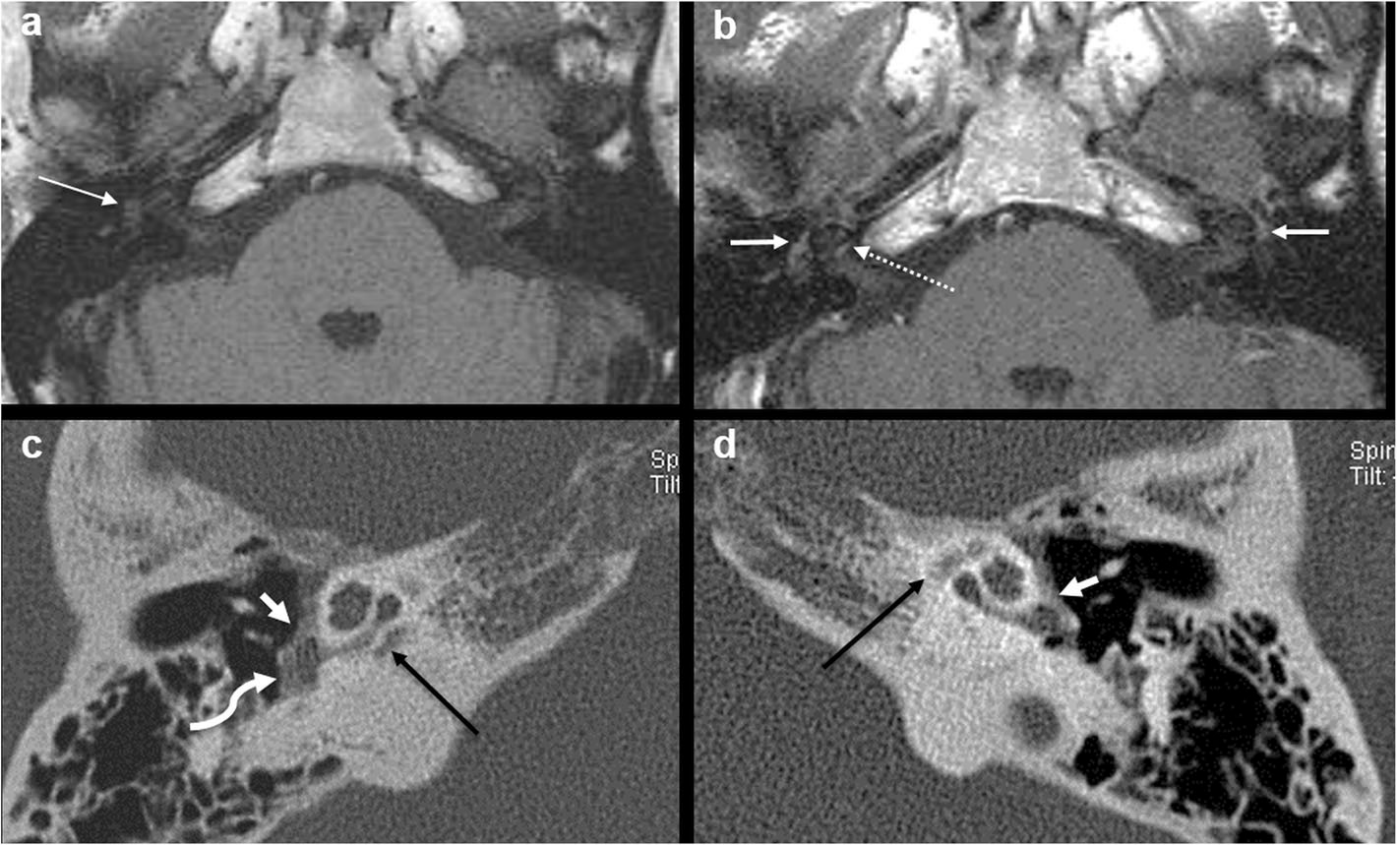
A 41-year-old male patient with left-sided SNHL (case 4). Axial T1W MR image (a) shows intermediate T1 signal in the right FA (arrow). Contrast-enhanced T1W MR image (b) shows moderate enhancement in bilateral FA regions (arrows) and mild enhancement in the right pericochlear region (dashed arrow). Pericochlear enhancement was not seen on the left. Subsequent axial HRCT images (c, d) confirm bilateral fenestral otosclerotic plaques (short white arrows) and pericochlear plaques (black long arrows). The right RW is occluded (curved arrow)
